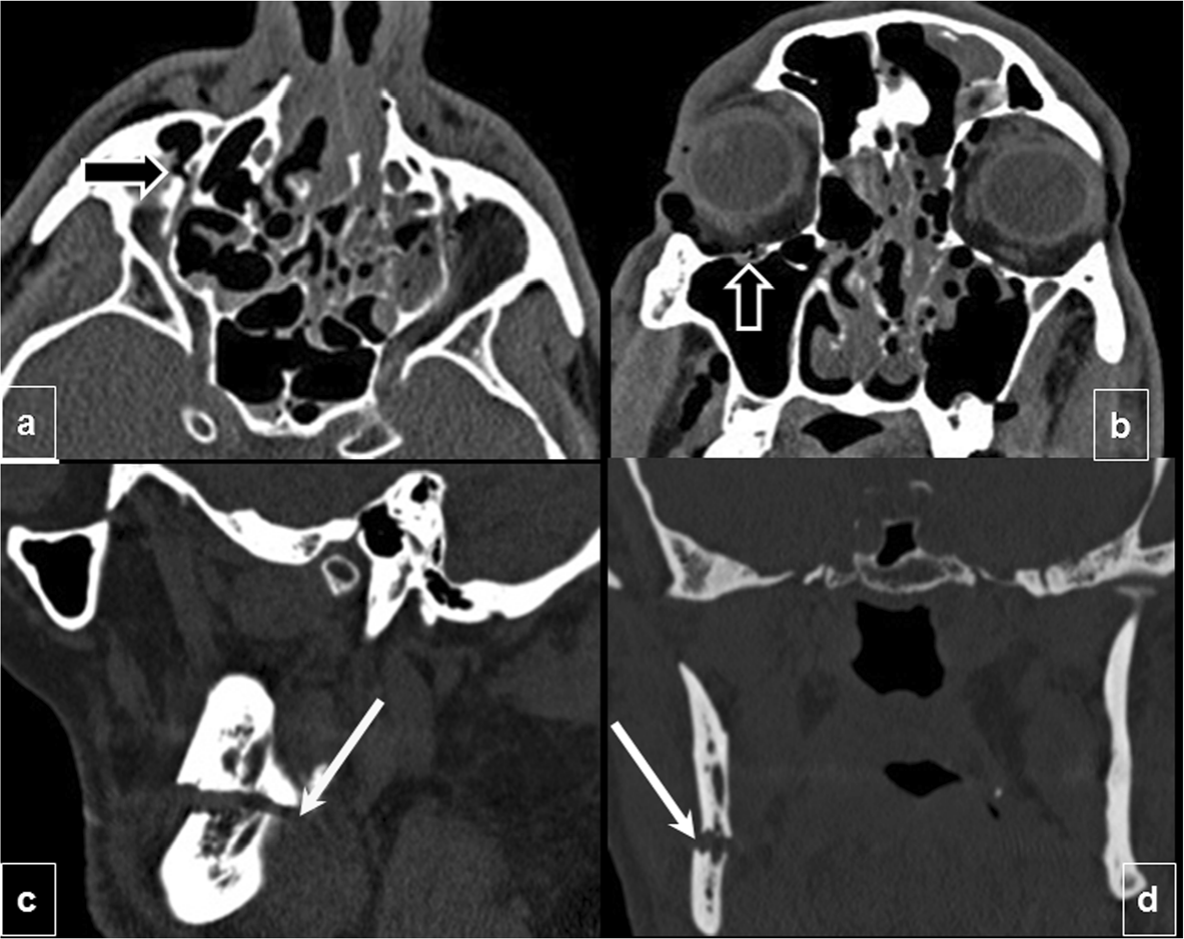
Neural foramina complications. Infraorbital canal injury: (a) Axial and (b) coronal CT images show a Le Fort II fracture passing through the infraorbital canal, which can affect the infraorbital nerve (terminal branch of CN V2) (black arrow). c, d Mandibular canal injury: (c) Sagittal and (d) coronal views of a unifocal mandibular fracture passing through the mandibular canal with mild displacement (white arrows) that may damage the inferior alveolar nerve (a branch of CN V3)
